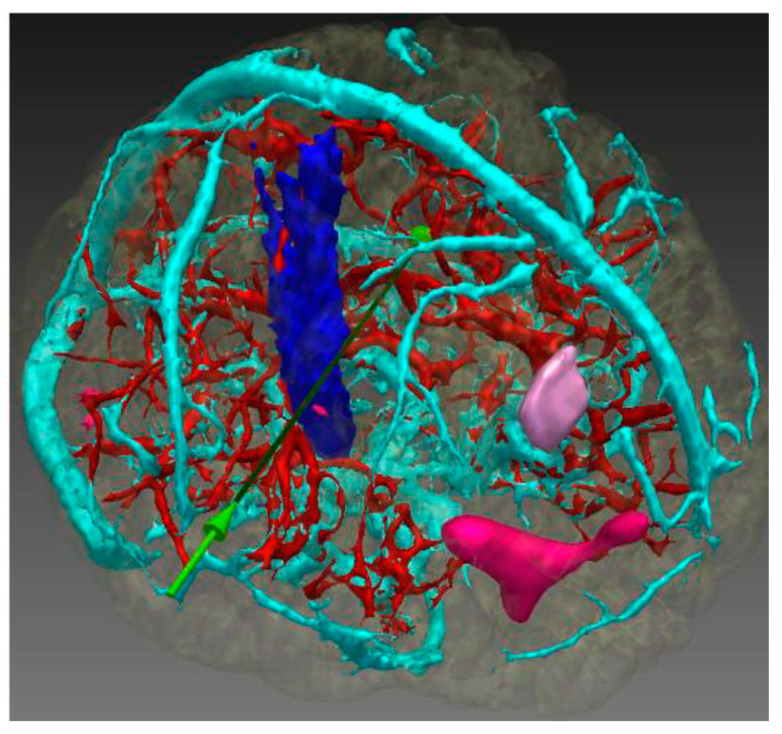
Path-planning example showing trajectory and critical structures. Blue: cerebrospinal tract (CST); pink: hypometabolism PET; deep pink: SPECT; cyan-red: blood vessels; green arrow: historical trajectory [16].
